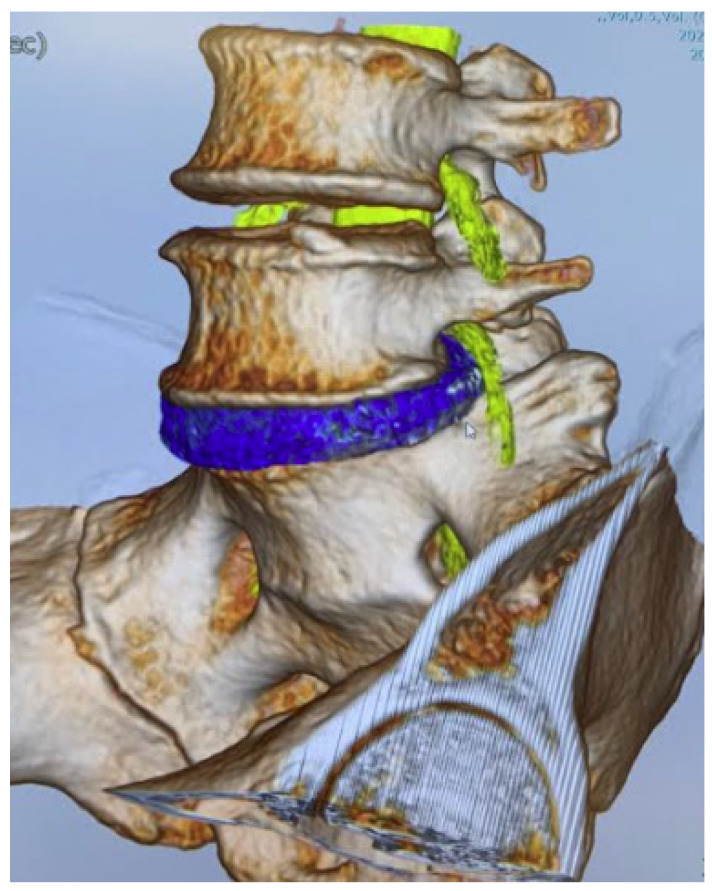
MRI and CT medical image fusion.
